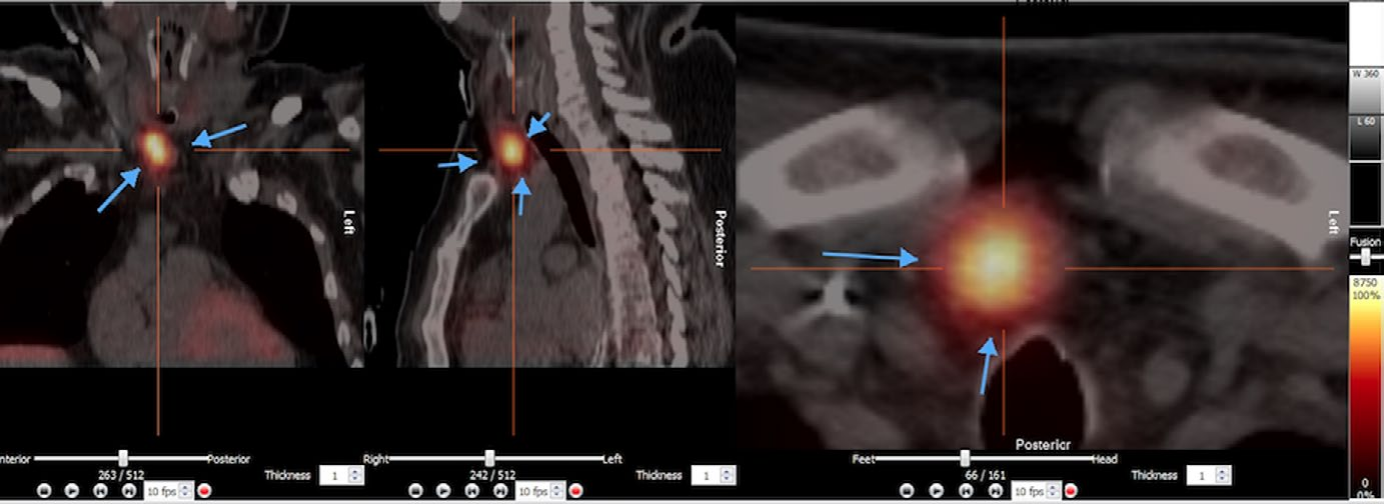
^99M^Tc‐sestamibi‐single photon emission computed tomography (SPECT) scan, showing a large adenoma of the right lower parathyroid gland
